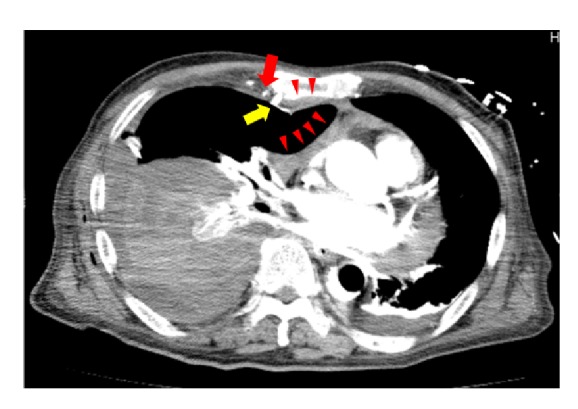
Contrast computed tomography (CT) scan on the next day of hospitalization. Right internal mammary artery (IMA) is divided into branches. A red arrow shows main branch of IMA and a yellow arrow shows the extravasation at a branch of IMA. There is small anterior mediastinal hematoma (red arrowheads) around the extravasation. Massive bloody pleural effusion is confirmed in the right lung.
